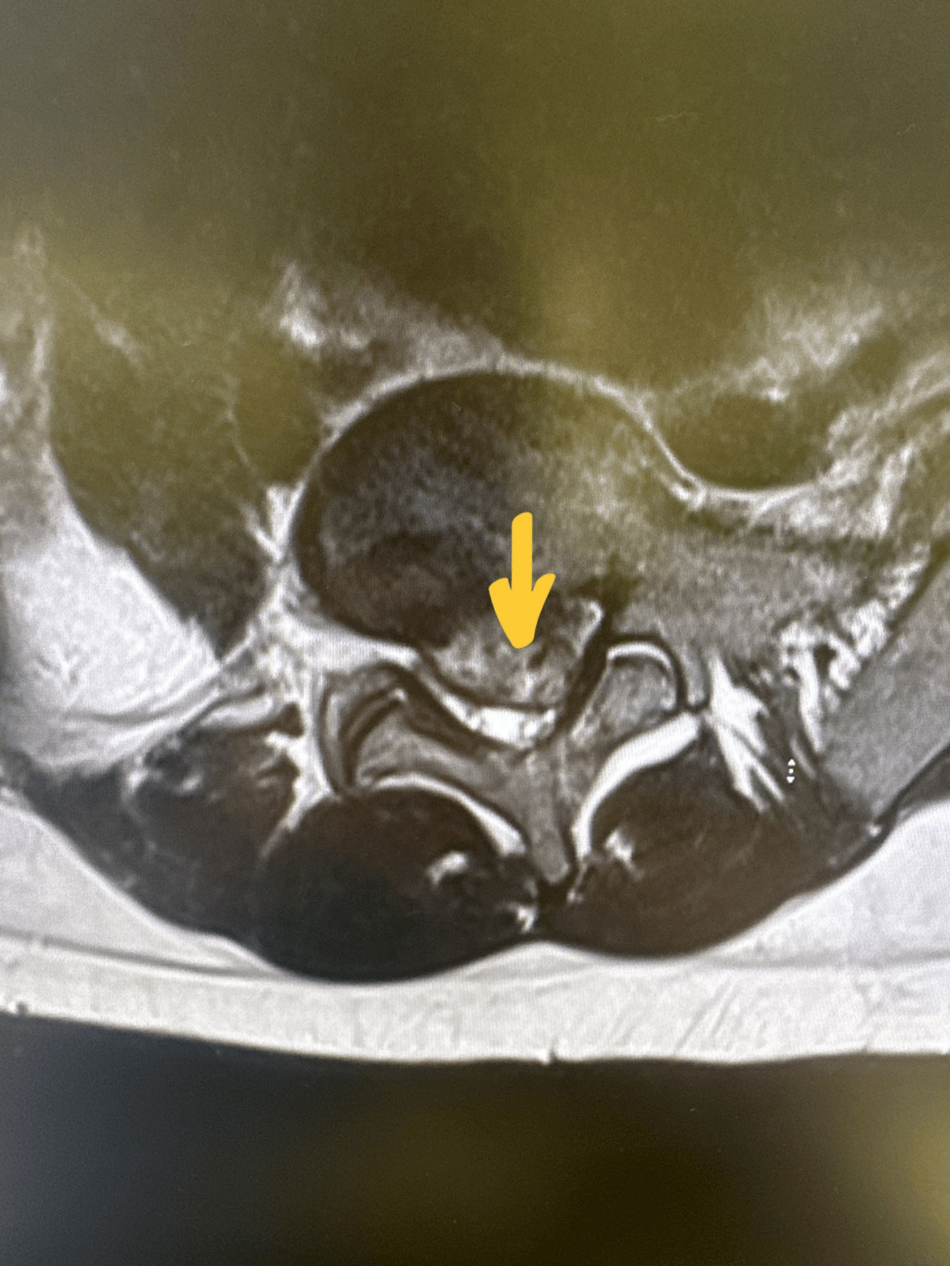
Sequential axial T2-weighted MRI image showing posterior disc protrusion (yellow arrow), causing severe spinal canal narrowing and bilateral recess stenosis.
